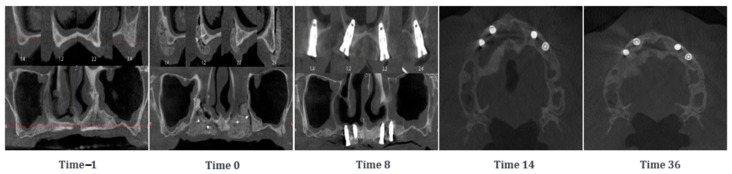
CBCT images of a 64-year-old woman with atrophy in the maxillary bone. SmartBone^®^ On-Demand plates were inserted, covering both the right and left alveolar processes of the upper arch. The images show the progression of bone healing over time, from pre-surgery (Time −1) to 36 months post-surgery (Time 36).
